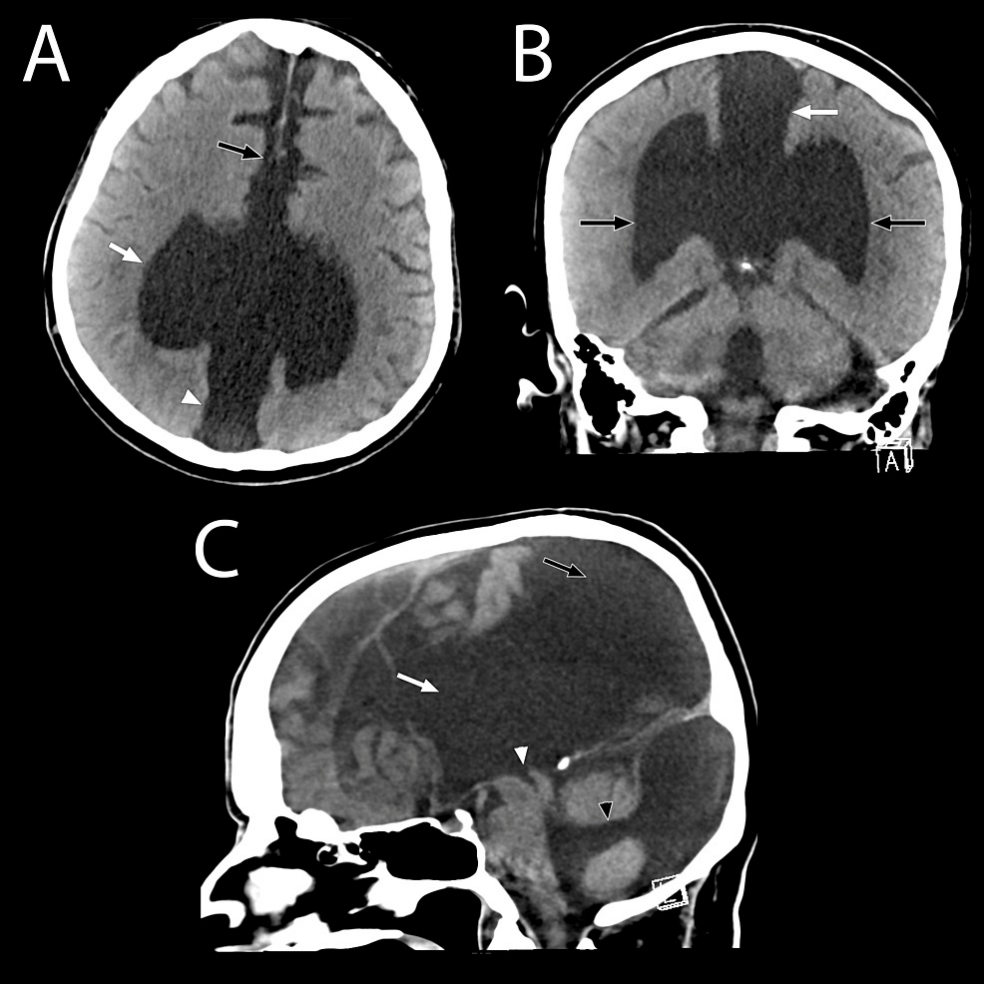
(A) Axial CT of the brain; (B) Coronal CT of the brain; (C) Midline Sagittal CT of the brain at the level of the medial longitudinal fissure. (A) The dorsal, midline, interhemispheric cyst (white arrowhead) is demonstrated along with its contiguous connection with the monoventricle (white arrow). A key feature identified in this view is the visibly separated frontal lobes (black arrow), with the presence of the falx cerebri, displaying non-fusion in the presence of an absent septum pellucidum. (B) The midline dorsal interhemispheric cyst (white arrow) communicates directly with the monoventricle. In this view, the monoventricle, which includes the third ventricle and the two lateral ventricles (indicated by black arrows), is clearly discernible. Note the clearly demarcated open communication between the dorsal interhemispheric cyst and the monoventricle, along with the absence of the corpus callosum. (C) The large monoventricle (white arrow) communicates with the dorsal interhemispheric cyst (black arrow) and is well appreciated. The Sylvian aqueduct draining the third ventricular component of the monoventricle is identified with the white arrowhead. The communication between the fourth ventricle (black arrowhead) and the posterior fossa cyst is evident in this view, in addition to the posterior fossa cyst. This view well demonstrates the essential components of the Dandy-Walker malformation and notes the absence of the corpus callosum.
